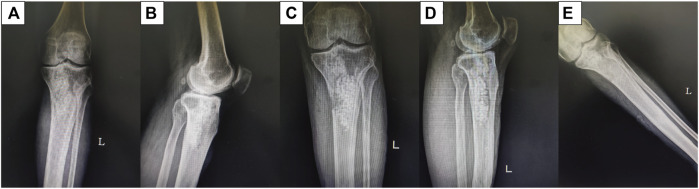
Male patient aged 43 years. Radiographs obtained before and after treatment with antibiotic-loaded calcium sulfate combined with autogenous iliac bone transplantation to manage proximal tibial osteomyelitis. (A, B) Preoperative anteroposterior and lateral position. (C, D) Anteroposterior and lateral position 1 d after the surgery. (E) Twelve months after the surgery.
